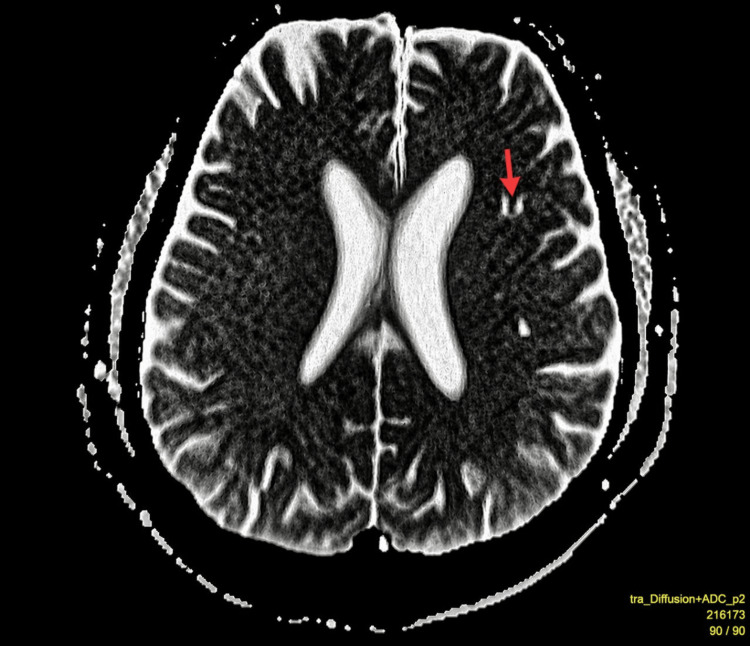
MRI of the brain An acute lacunar infarct is noted in the left external capsule. Subtle diffusion/FLAIR mismatch is present. MRI: magnetic resonance imaging; FLAIR: fluid-attenuated inversion recovery
